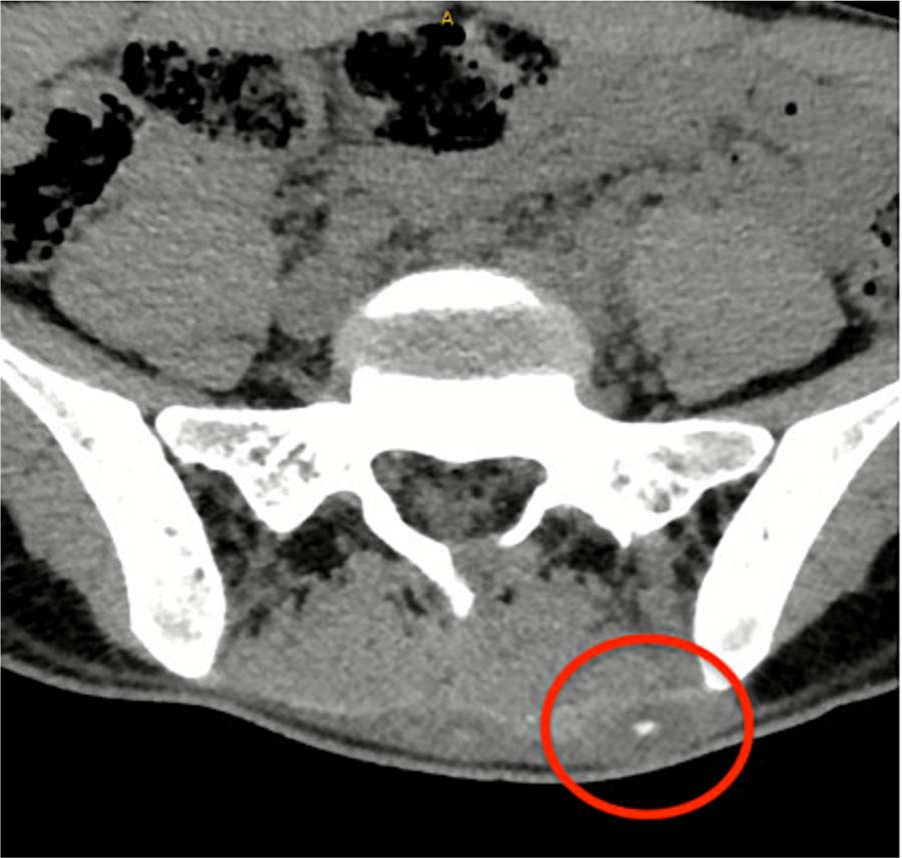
CT lumbosacral spine showed a left paraspinal calcified haematoma.
